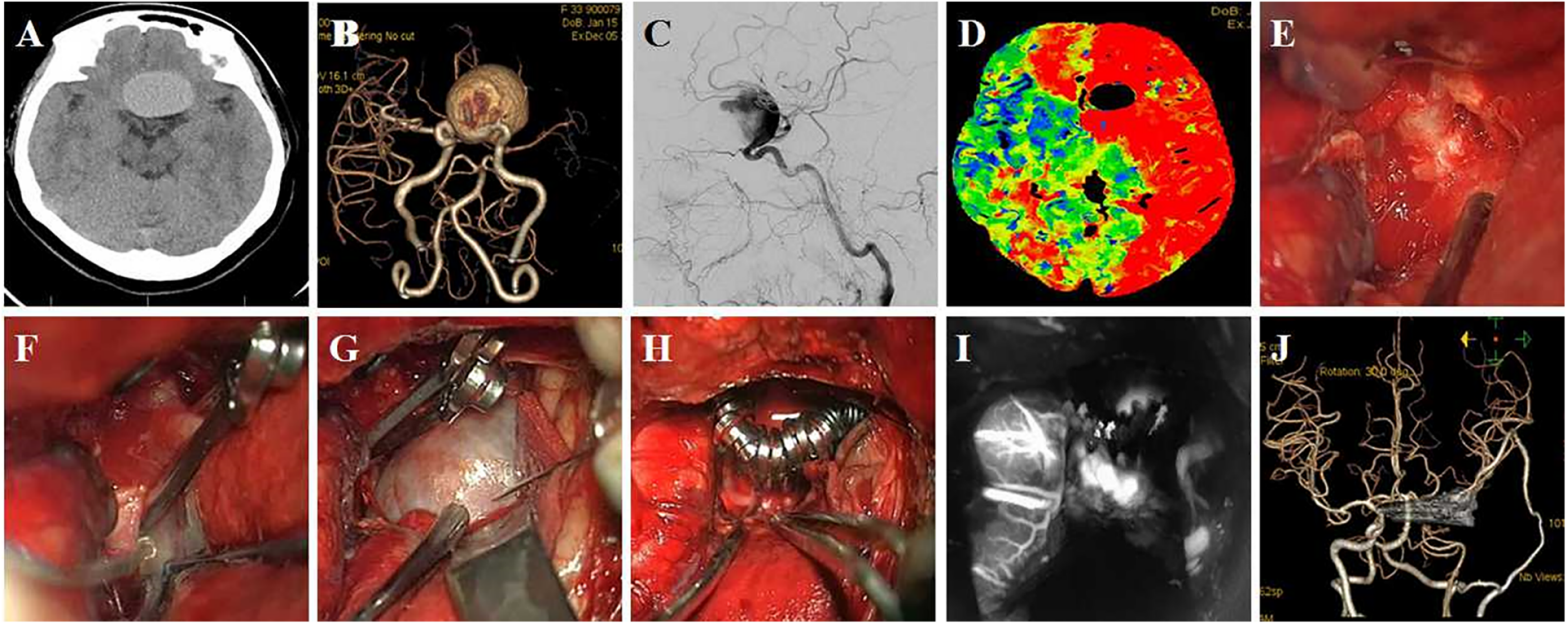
A 33-year-old female patient presented with recurrent headache, which had lasted 1 year. (A) Preoperative CT shows a sellar space-occupying lesion; (B,C) CTA and DSA show a giant C6 aneurysm and non-visualization of ipsilateral MCA; (D) CTP shows hypo-perfusion on ipsilateral ICA distribution area; (E) exposed clinoid segment of ICA and the distal ring was seen; (F) difficulty in clipping aneurysm neck due to calcification; (G) aneurysm puncture after temporary occlusion; (H) the aneurysm was clipped by multiple clips; (I) ICG in surgery shows disappearance of aneurysm and patency of parent artery; (J) postoperative CTA shows absence of the aneurysm, and superficial temporal artery-middle cerebral artery anastomosis is patent.
